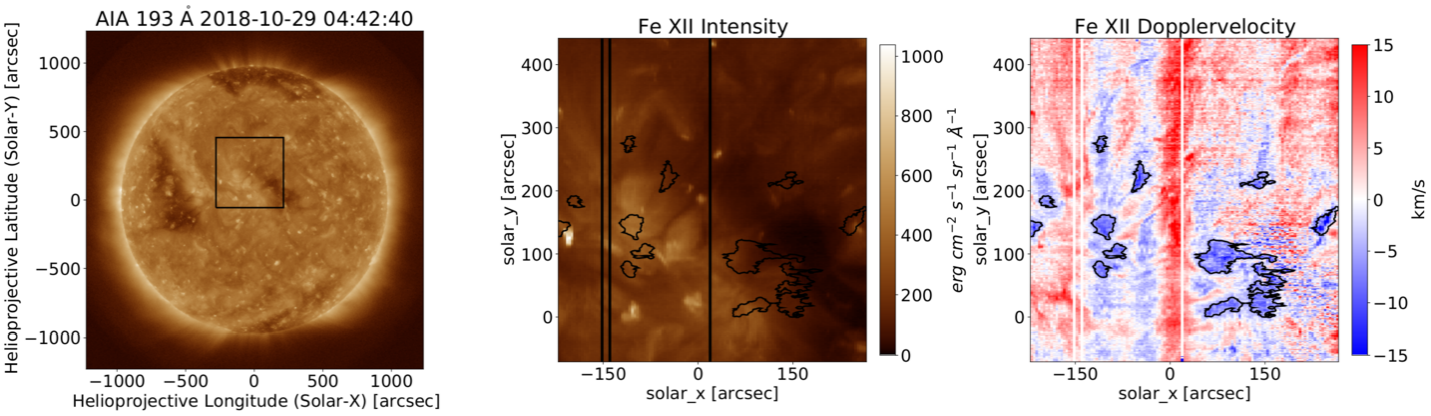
A Hinode/EIS raster of the small CH on the 29 October 2018 at 04:42:42 UT, 195.12 Å. The *left panel* shows the EIS field-of-view on the disc. The *central panel* shows the intensity of the Fe xii line. The *right panel* shows the Doppler velocities derived from the Fe xii line. 13 regions of blueshifts stronger than -5 km s^−1^ are highlighted. They are equally distributed at the small CH boundary and on the quiet Sun.
